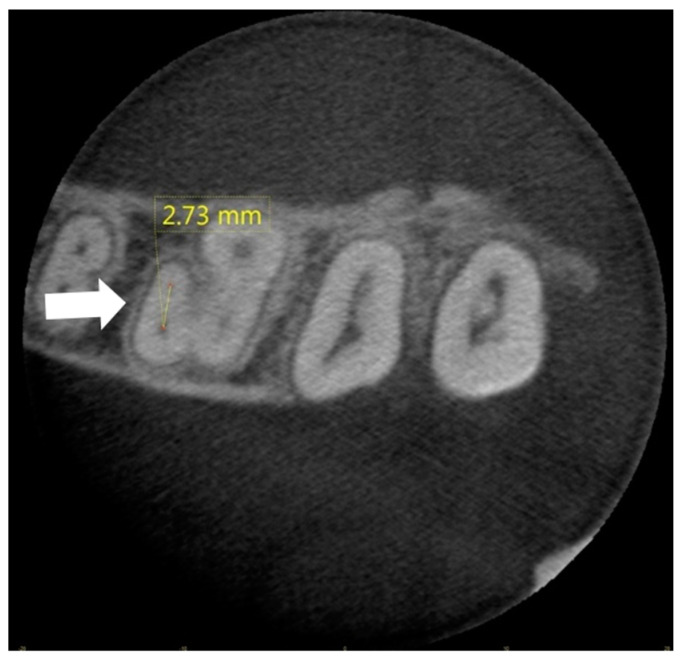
CBCT reconstructions of MB root in maxillary first molar tooth. First axial section in which MB2 canal was visualized. IOD was measured between MB1 and MB2 canals.
